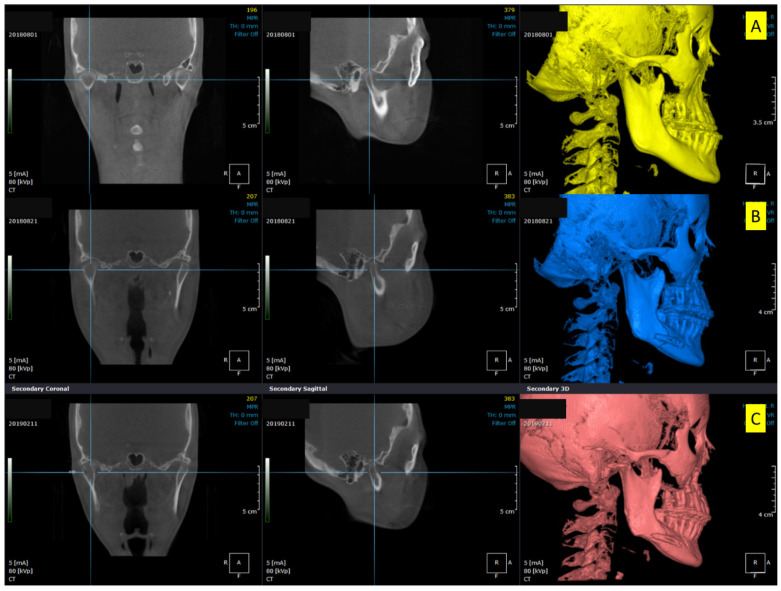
Three-dimensional cone-beam computed tomography scans showing the changes in condylar positions in Patient no. 2. (A) Pre-surgical, (B) Post-surgical, (C) Five months after screw removal. Increased temporomandibular joint space is seen in (B). After screw removal, the joint space decreased and was restored to the pre-surgical state.
